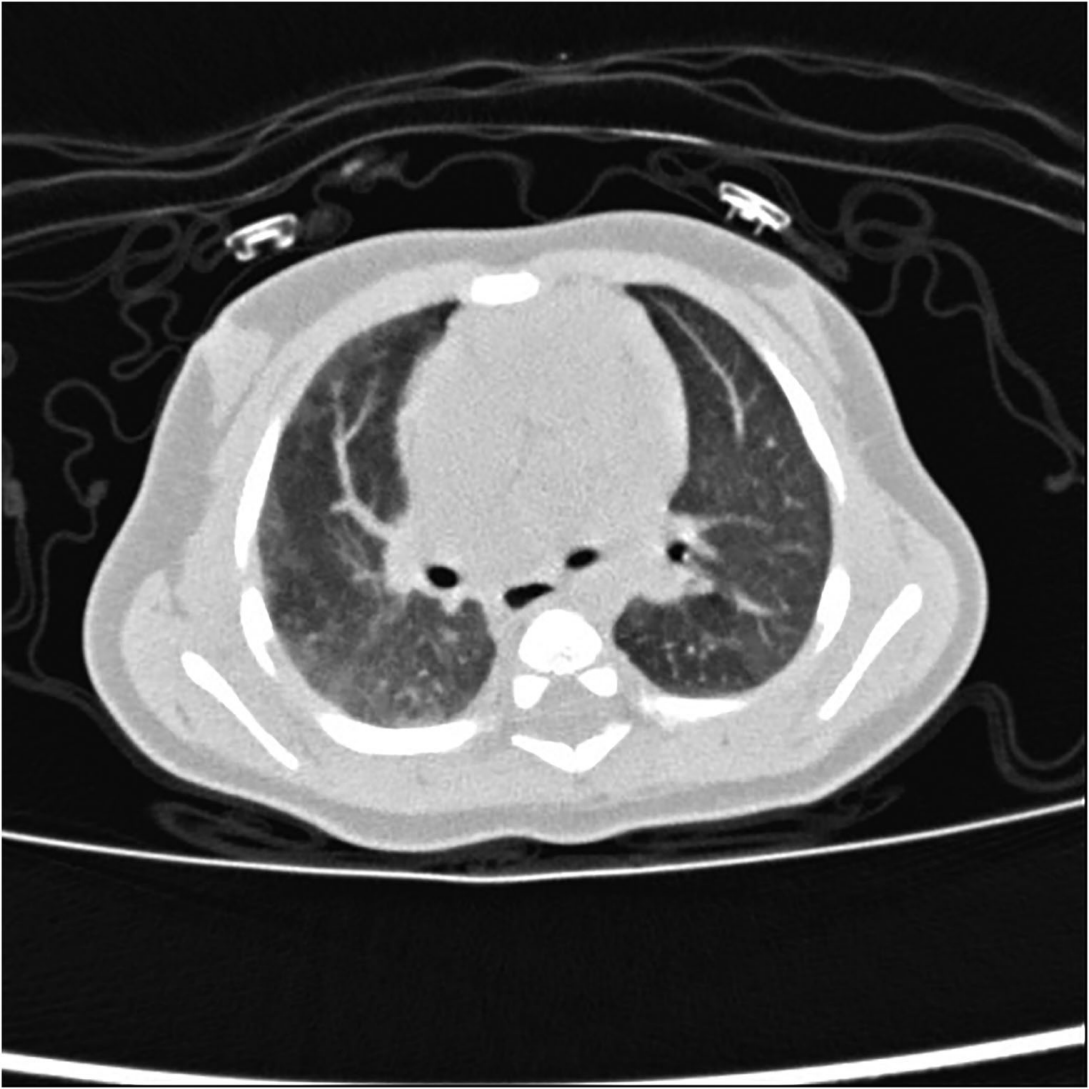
Right proximal interruption of the pulmonary artery in a 1-month-old male patient. A transverse view of a plain computed tomography scan shows strip-like shadows of subpleural pulmonary parenchyma along the periphery of the right lung at ground-glass opacity and a mild mosaic sign in the left lung.
